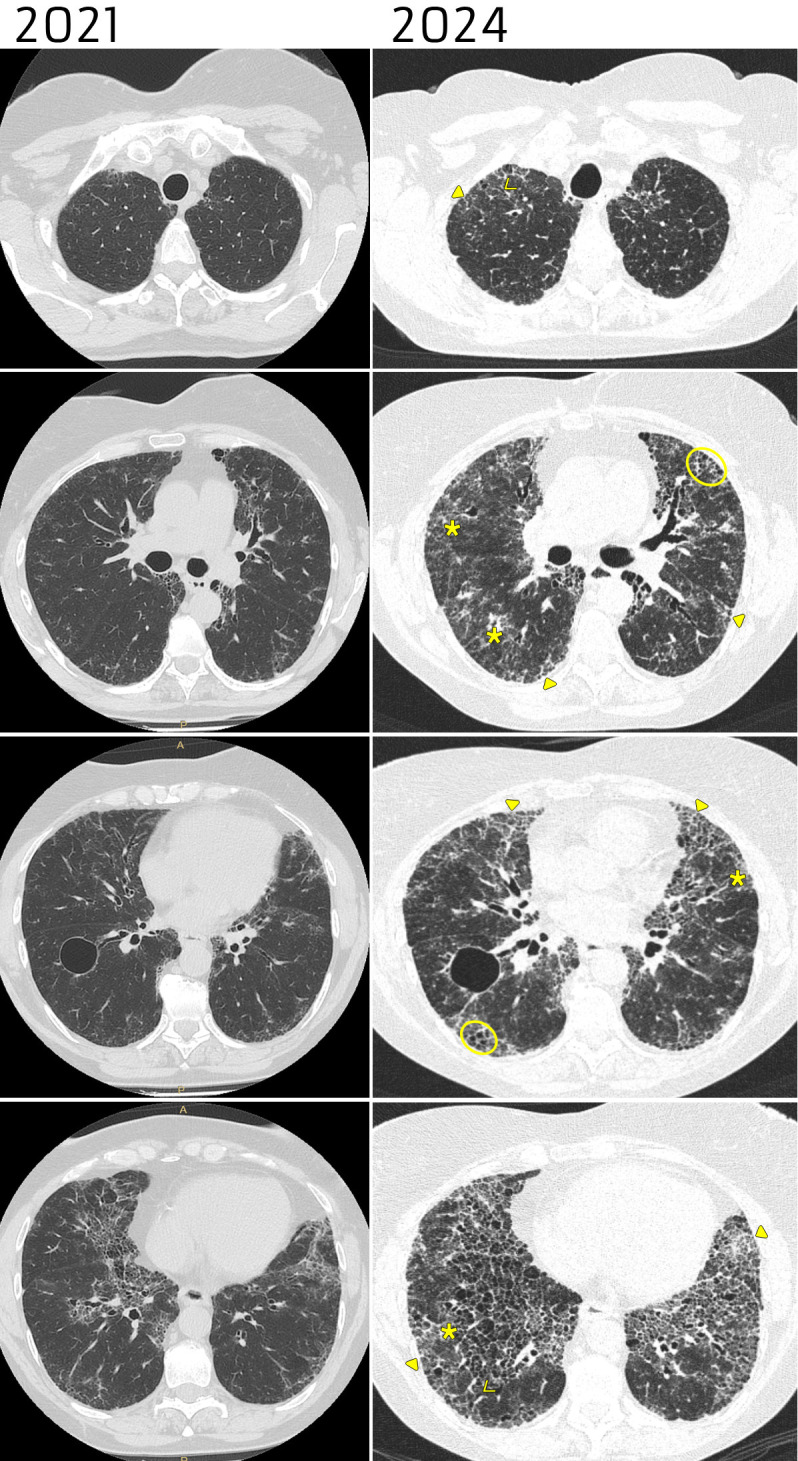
HRCT of the chest showing radiological progression from 2021 to 2024, with increased ground glass opacities and fibrosis. The 2024 scans show fibrosis characterized by reticular thickening of the interstitium (triangles), mild ground-glass opacities (asterisks), multiple traction bronchiectasis (arrows), and honeycombing (circles) with apicobasal gradient, configuring a UIP pattern.
